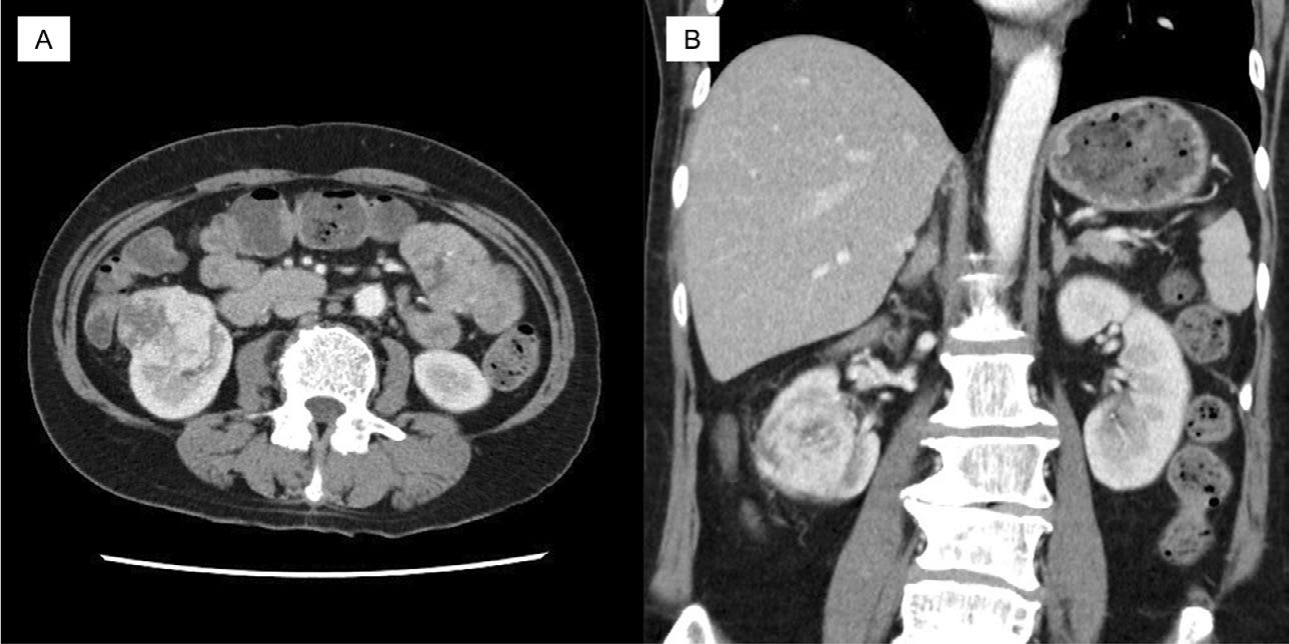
Contrast‐enhanced CT showed an approximately 5 cm renal tumor with contrast effect on the lower pole of the kidney. (A) Axial section (B) Coronal section.
